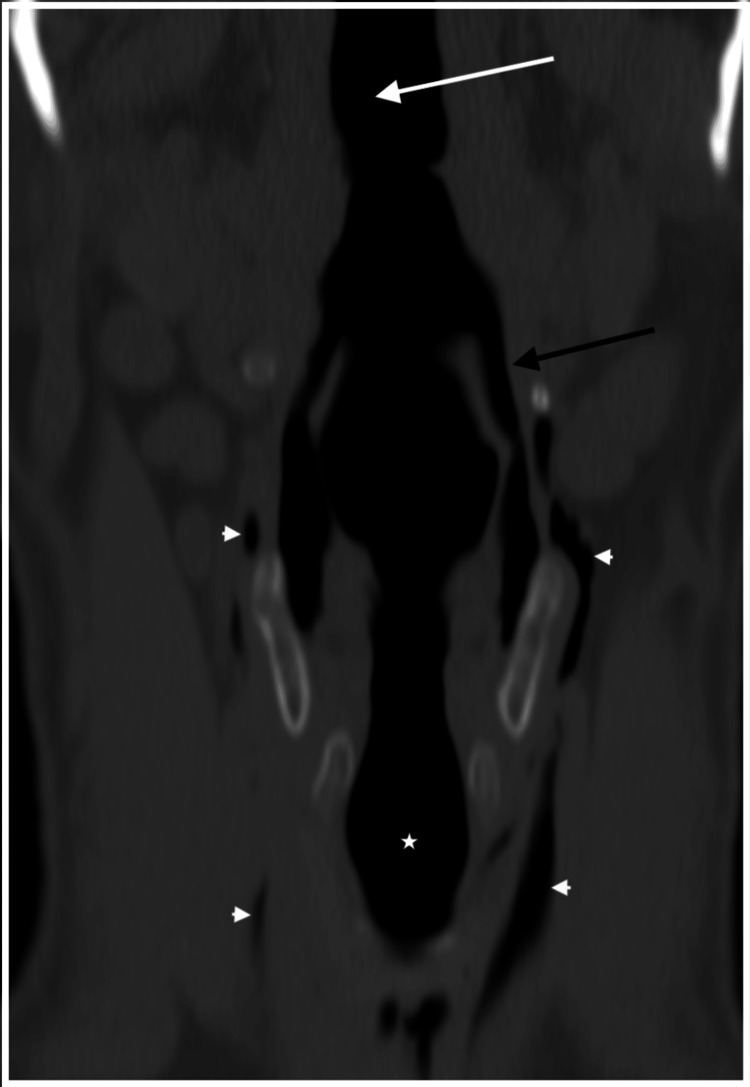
Bone window coronal CT of the neck. Aero-digestive tract (white arrow), proximal trachea (*), pyriform sinus (black arrow), and emphysema in the visceral space (arrowheads).
